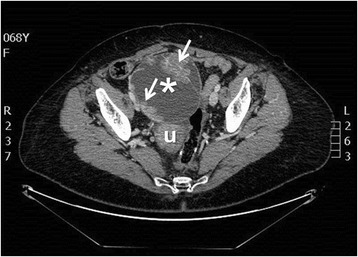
Contrast-enhanced CT scan showing a complex and heterogeneous pelvic mass with inner vegetations and septations in the anatomic site of the right adnexa (max diameter 17 cm), not dissociable from uterus and contiguous bowel (asterisk: pelvic mass; arrows: vegetations and septations; u: uterus).
